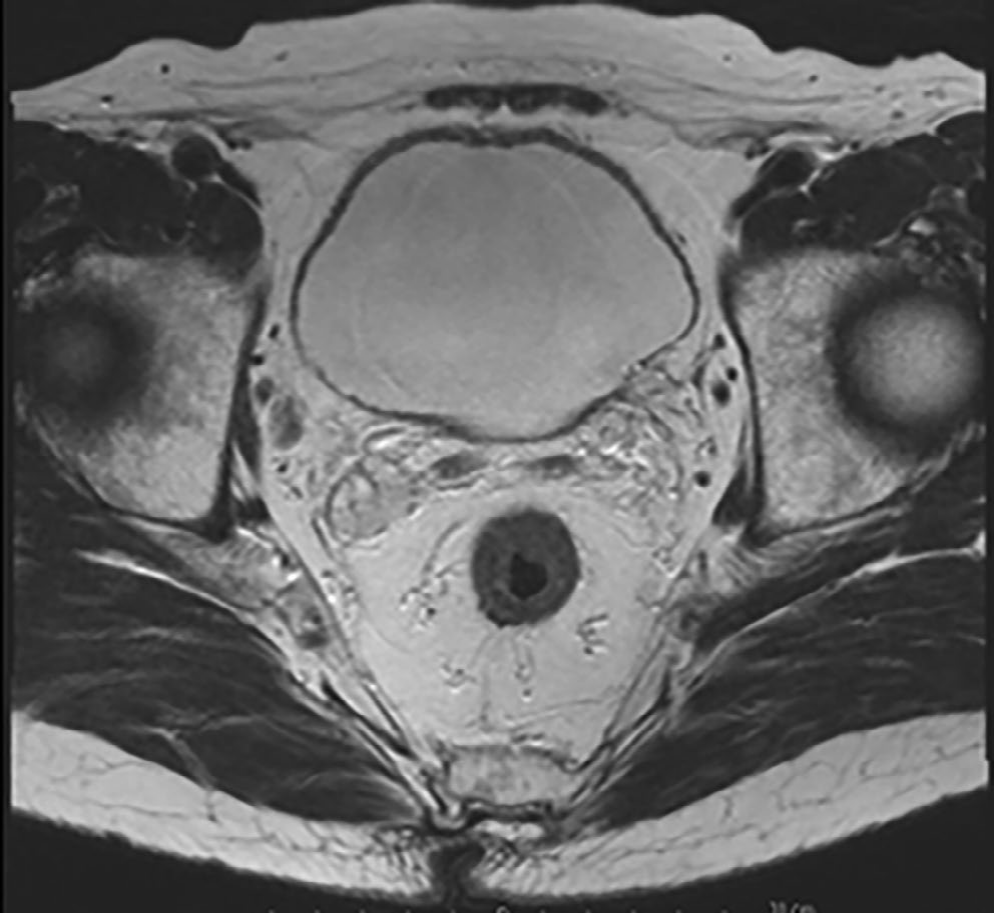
T2‐weighted image of MRI after resection of IDBT and fulguration of the isolated diverticulum.
